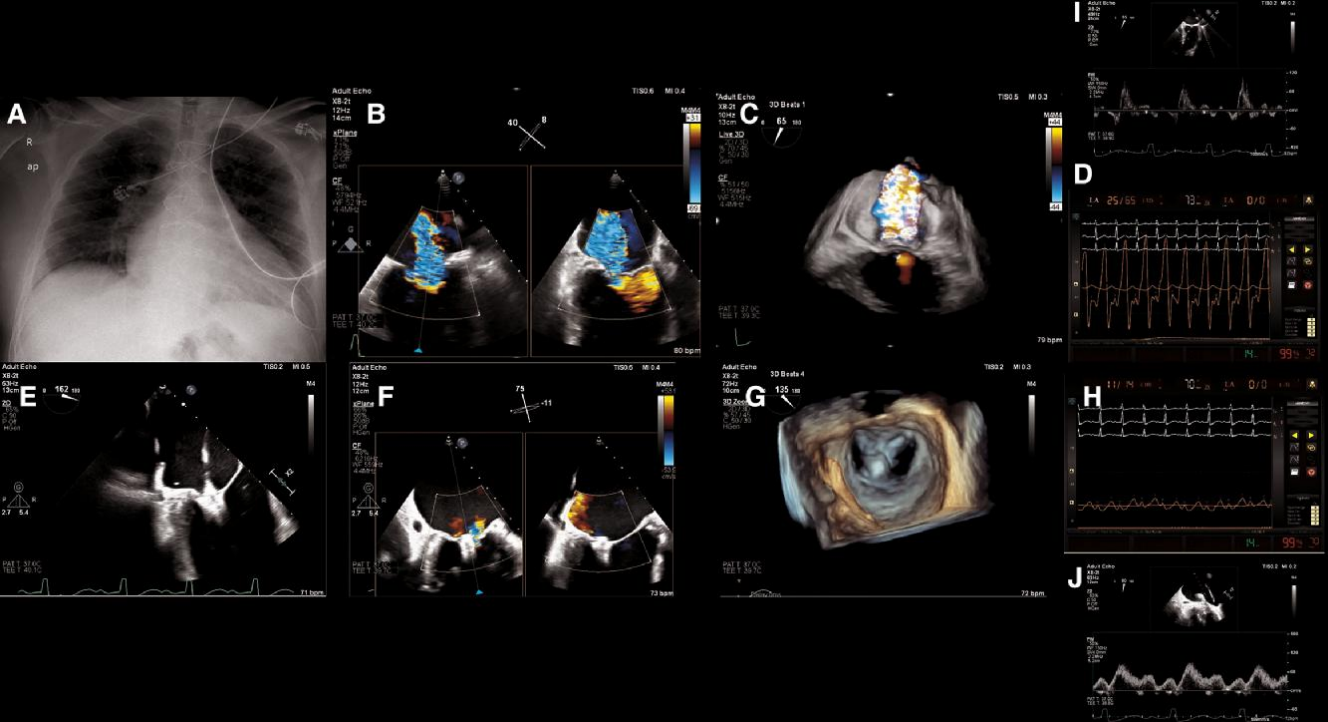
(*A*) Admission chest radiograph (posteroanterior view). Cardiomegaly with pulmonary congestion and interstitial pulmonary oedema. (*B*) Midesophageal commissural biplane view with colour flow Doppler. Wide vena contracta of mitral regurgitation measuring 32 mm. (*C*) Live 3D colour from a commissural view. Severe mitral regurgitation from across A2-P2 area. (*D*) Left atrial haemodynamic tracing upon entry. Giant V waves of 65 mmHg, indicative of severe mitral regurgitation. (*E*) Leaflet clasping with Pascal device. (*F*) Final result in biplane imaging with colour flow Doppler. (*G*) Three-dimensional enface view of the mitral valve after Pascal implantation. Double orifice valve. (*H*) Final left atrial haemodynamics after Pascal implantation. V waves of 14 mmHg. (*I*) Pulmonary venous flow before TEER. Systolic flow reversal. (*J*) Pulmonary venous flow post-TEER. Systolic S wave predominance.
